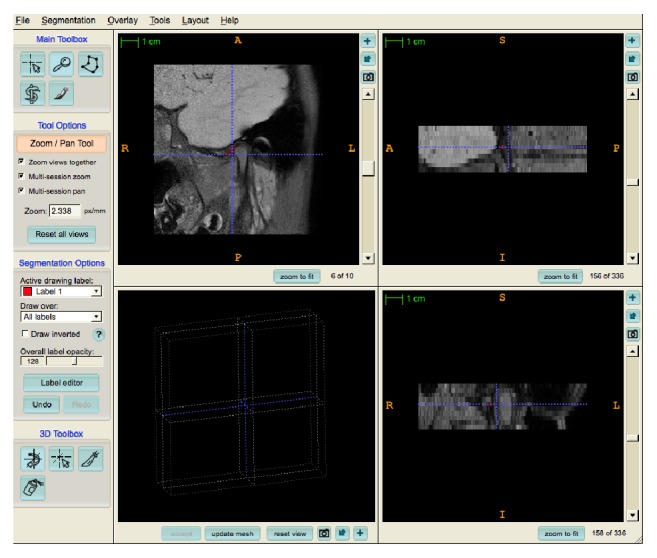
Software interface showing delimitation of the articular eminence in the sagittal plane on MRI.
